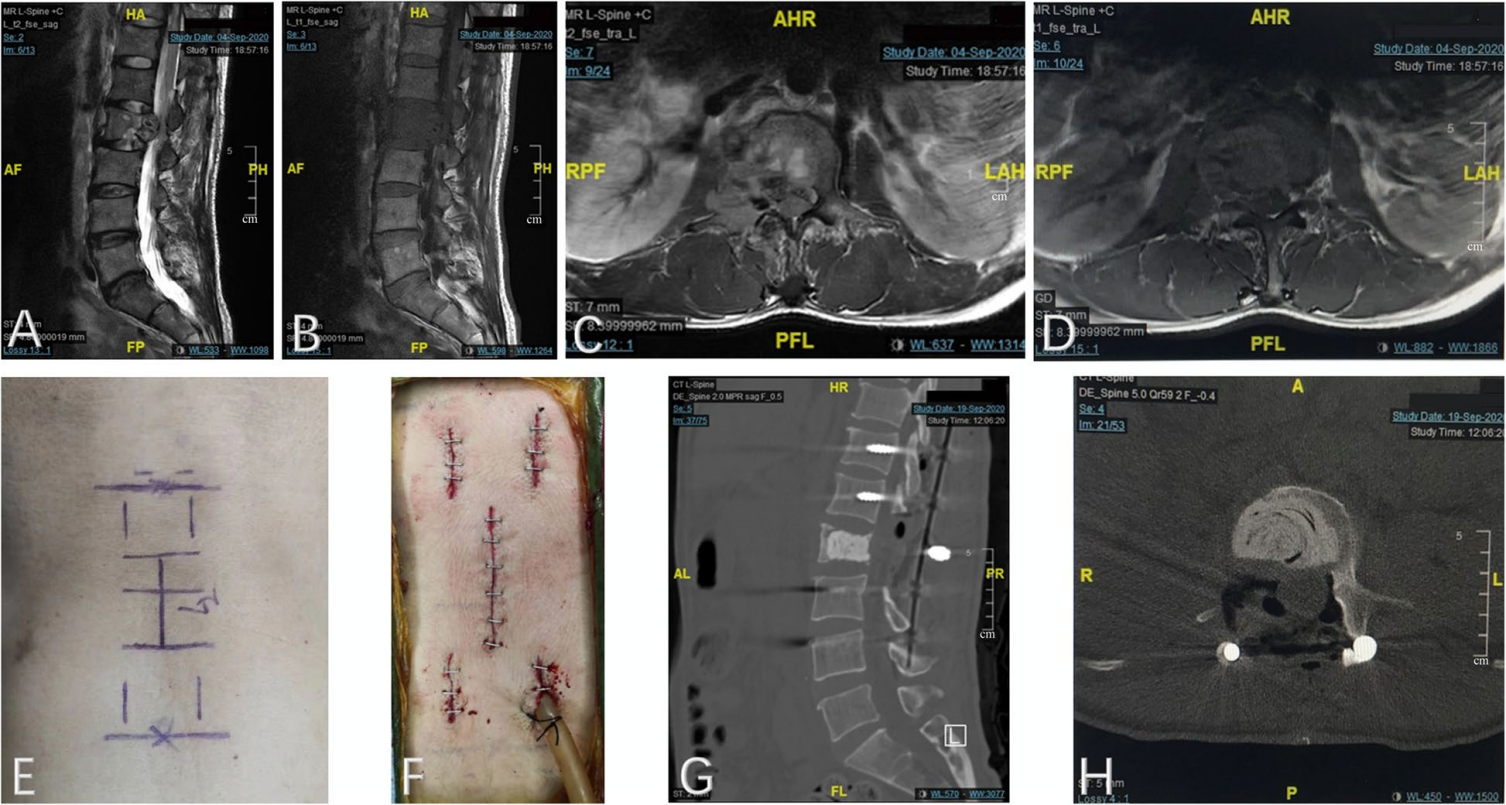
A representative case: a 52-year-old man with L2 hepatic carcinoma metastasis. A-D The T2and T1-weighted sagittal (A and B) and axial (C and D) MRI images showed a destructive lesion involving L2 with high-grade ESCC (Grade 2). E–F Photographs showing the 5–6 cm incision in the midline for tumor debulking and spinal cord decompression (E). Another four small incisions (1.5-2 cm) were made for pedicle screws and rods implantation (F). G-H The sagittal (G) and axial (H) CT images demonstrated pedicle screws and rods position and PMMA after minimally invasive separation surgery (MISS)
